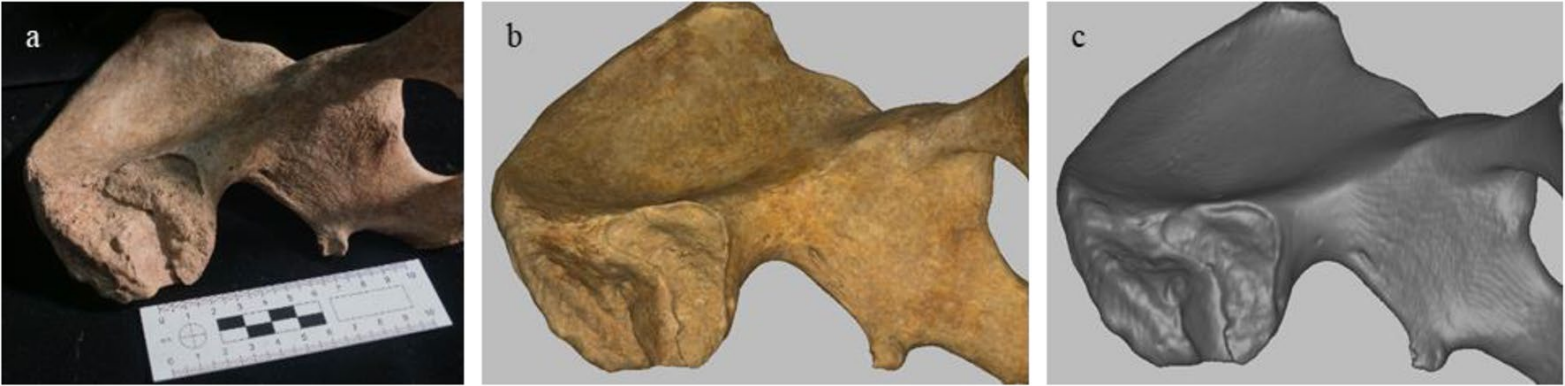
Left coxal bone of Ins Kirchgemeindehaus individual 3543 on a dry bone, b Artec 3D surface scan, and c CT scan
